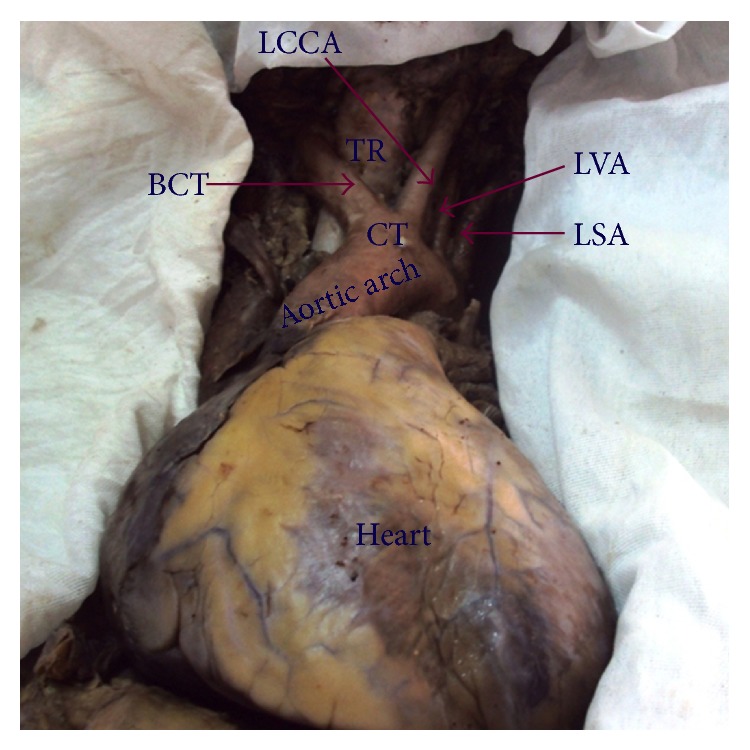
Aortic arch showing three branches (common trunk for brachiocephalic trunk and left common carotid artery). CT: common trunk, BCT: brachiocephalic trunk, LCCA: left common carotid artery, LSA: left subclavian artery, LVA: left vertebral artery, and TR: trachea.
